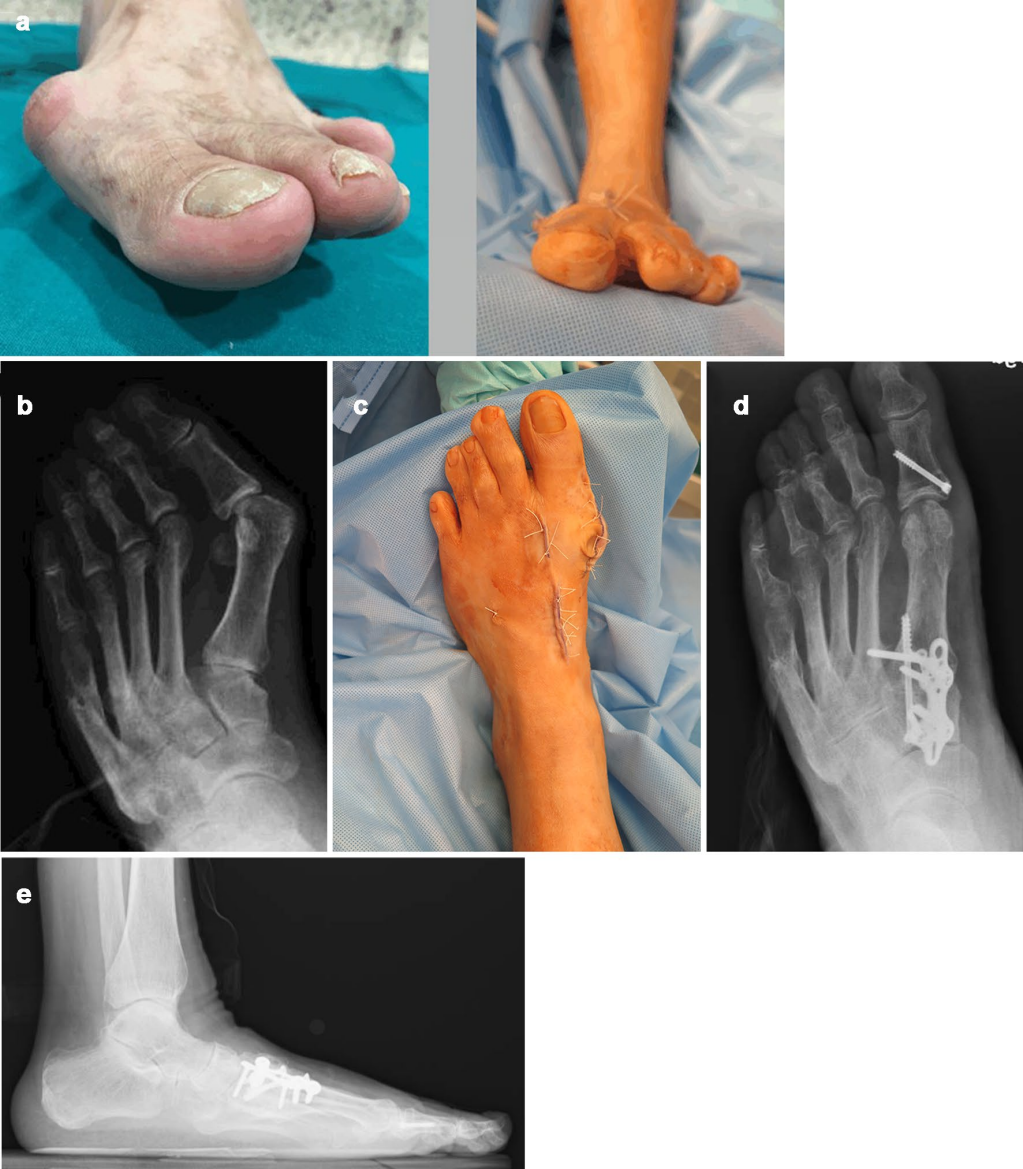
A 61-year-old male with severe TMT hypermovility and hallux valgus. a Note the preoperative pronation of the hallux and the postoperative correction; b Anteroposterior X-ray, note a consolidated stress fracture of the second and fifth metatarsal bones; c Clinical view after the operation; d, e Control X-rays at 12 months postoperatively
